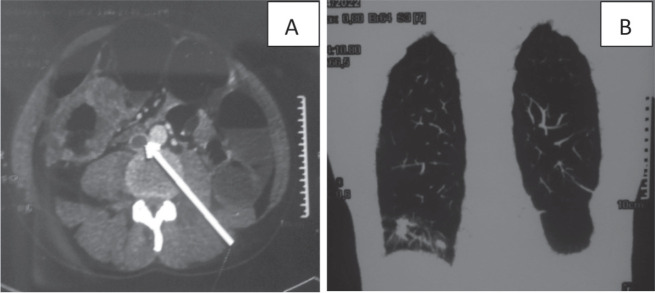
CT scan of a 37-year-old man: (a): Cross-section of inferior vena cava thrombosis (white arrow) and a circumferential mass of the right colon. (b): Frontal section of lung metastases.
